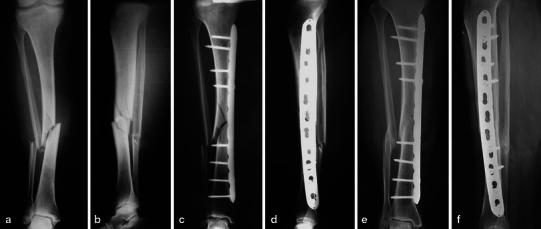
Preoperative anteroposterior (a) and lateral (b) radiographs of 56-year-old female patient show a wedge type (AO 42-B1) right tibia fracture and associated fibula fracture at the same level. Tibia fracture was fixed with a 4.5 mm locking compression plate (c and d). One proximal screw hole close to the fracture was omitted. Plate-span ratio was 4.6 and plate-screw density was 0.66. 18 months postoperatively, anteroposterior (e) and lateral (f) radiographs showed uneventfully healing of the fracture without any complications
